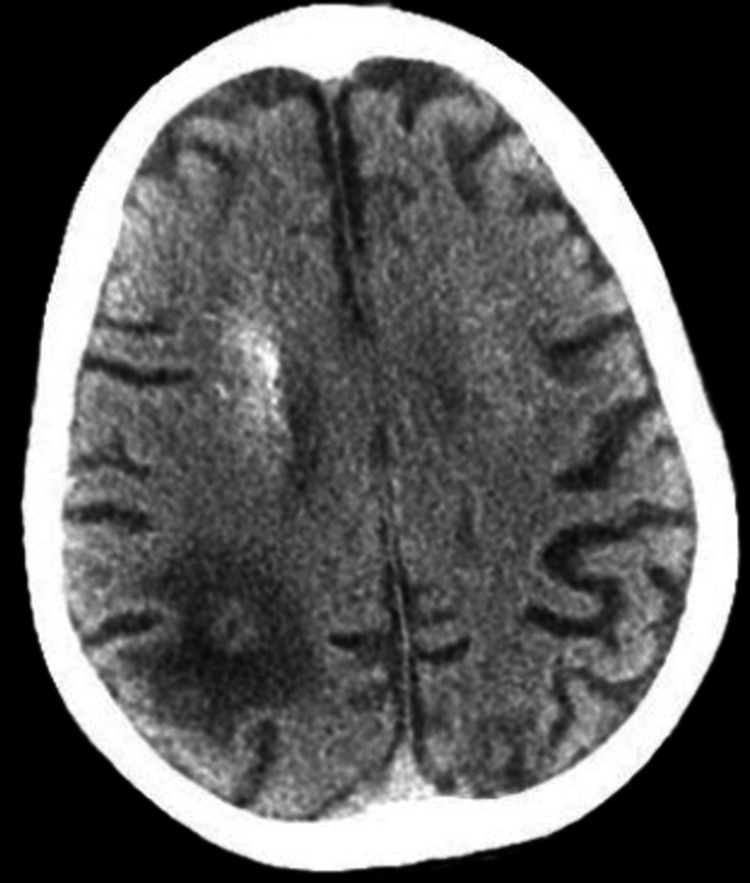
Non-Contrast CT of the brain, axial view This image demonstrates a large hypodense lesion at the right occipito-parietal lobe, showing an old infarct.
